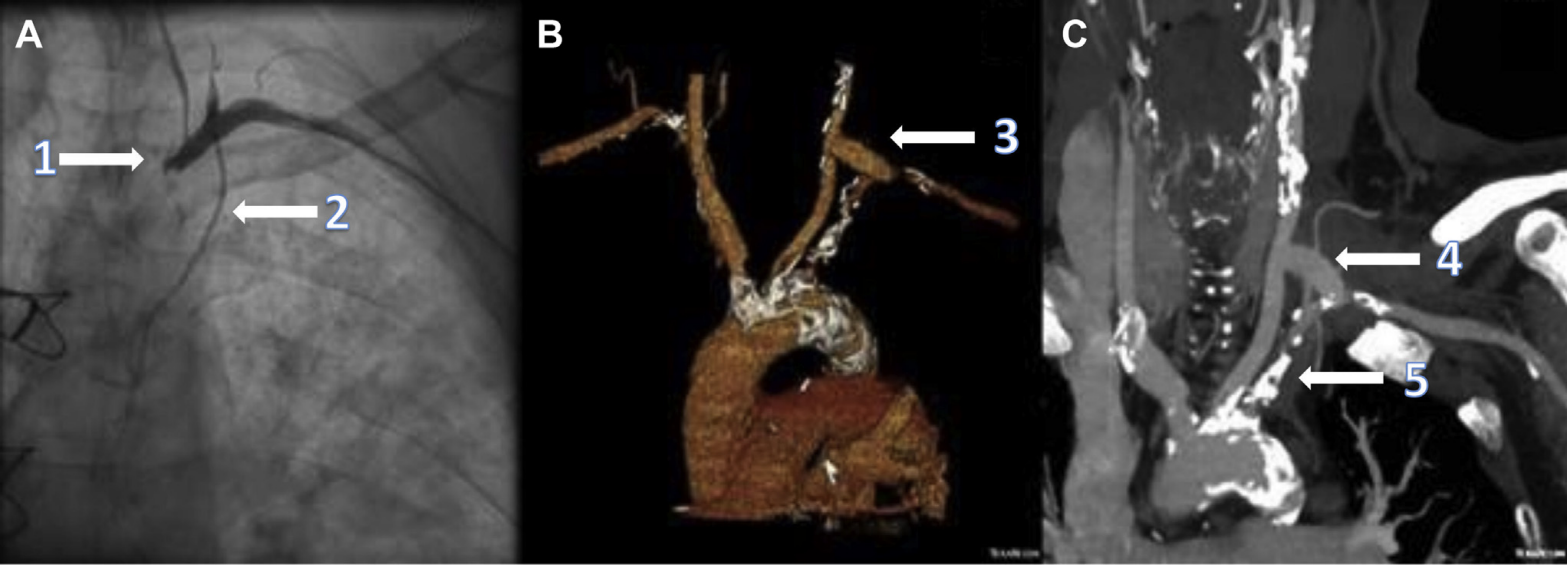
Cardiac catheterization, with an (A) anteroposterior view demonstrating complete occlusion of the (1) left subclavian artery (LSCA) and (2) patent left internal mammary artery graft, (B) postoperative cardiac computed tomography angiography with reconstruction showing the (3) newly implanted graft from the left carotid artery to LSCA, and (C) coronal view demonstrating the (4) patent graft from the left carotid artery to LSCA. Note the (5) extensive atherosclerotic disease in the origin of the LSCA.
